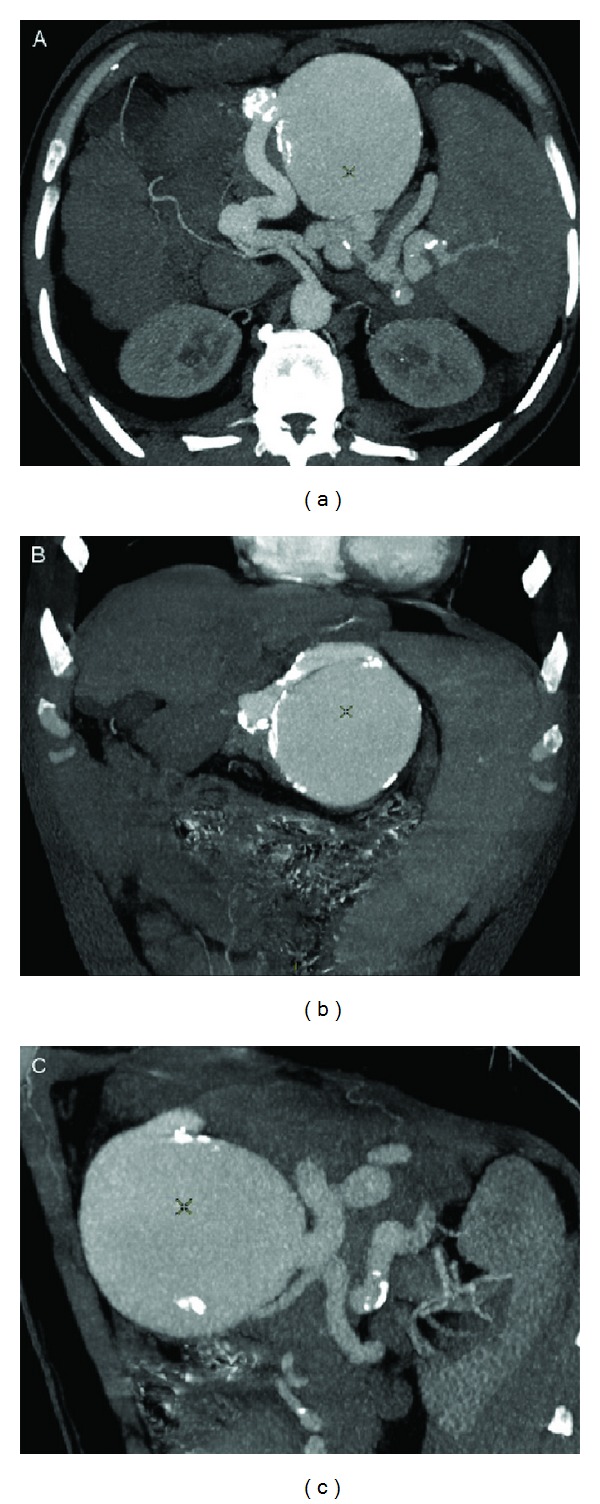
Computed tomography (CT) of the abdomen showing a tortuous and ectasic splenic artery with some widely dilated sections (max 24 mm calibre) and massive aneurysmal dilation in the middle tract (90 mm of maximum diameter) with partly calcified walls, inducing a cranial displacement of the tail and the body of the pancreas.
